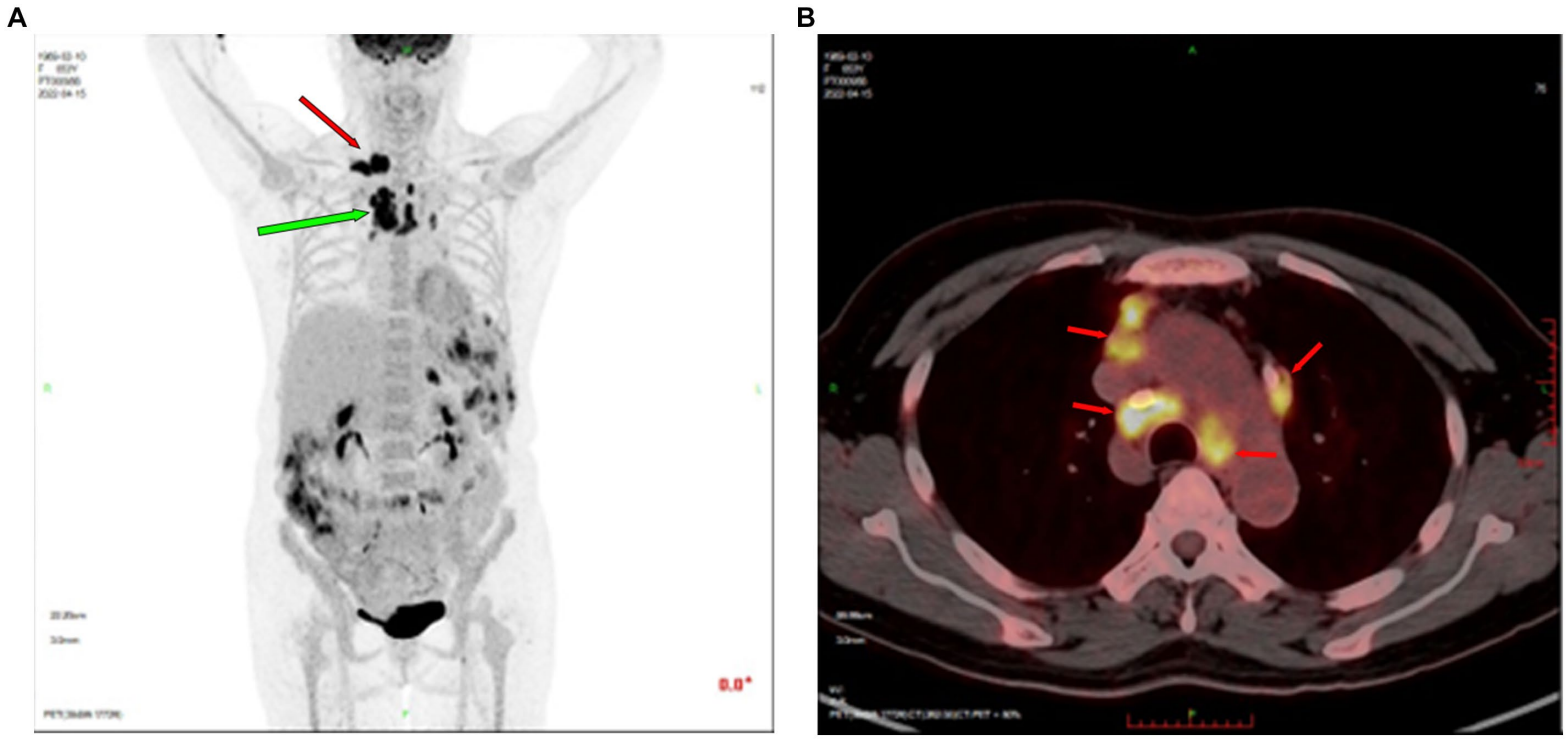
Positron emission tomography-CT revealed excessive FDG accumulation in multiple lymph nodes. (A) The right supraclavicular lymph node, approximately 27 mm in diameter, showed increased FDG uptake (SUVmax = 15.6, indicated by red arrow). Enlarged mediastinal lymph nodes showed increased FDG uptake (green arrow). (B) Excessive FDG accumulation in mediastinal lymph nodes (red arrow). CT, computed tomography; FDG, fluorodeoxyglucose; SUVmax, maximum standard uptake value.
